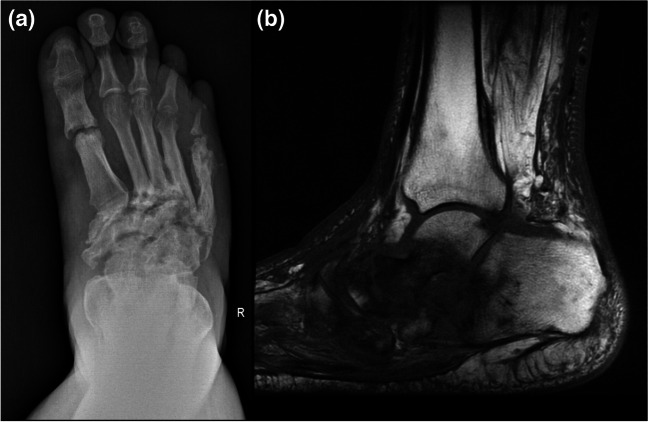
Anteroposterior radiograph of the foot (a) shows destruction and deformity of the tarsal and metatarsal bones with sclerosis, joint space narrowing, cortical irregularities, and fragmentation, Lisfranc injury involving the second to fifth tarsometatarsal joints with lateral dislocation in a patient with neuropathic osteoarthropathy. Sagittal T1W MR image (b) reveals a collapse in the longitudinal arch of the foot with a generalized bone marrow edema throughout the tarsal and metatarsal bones in the midfoot
